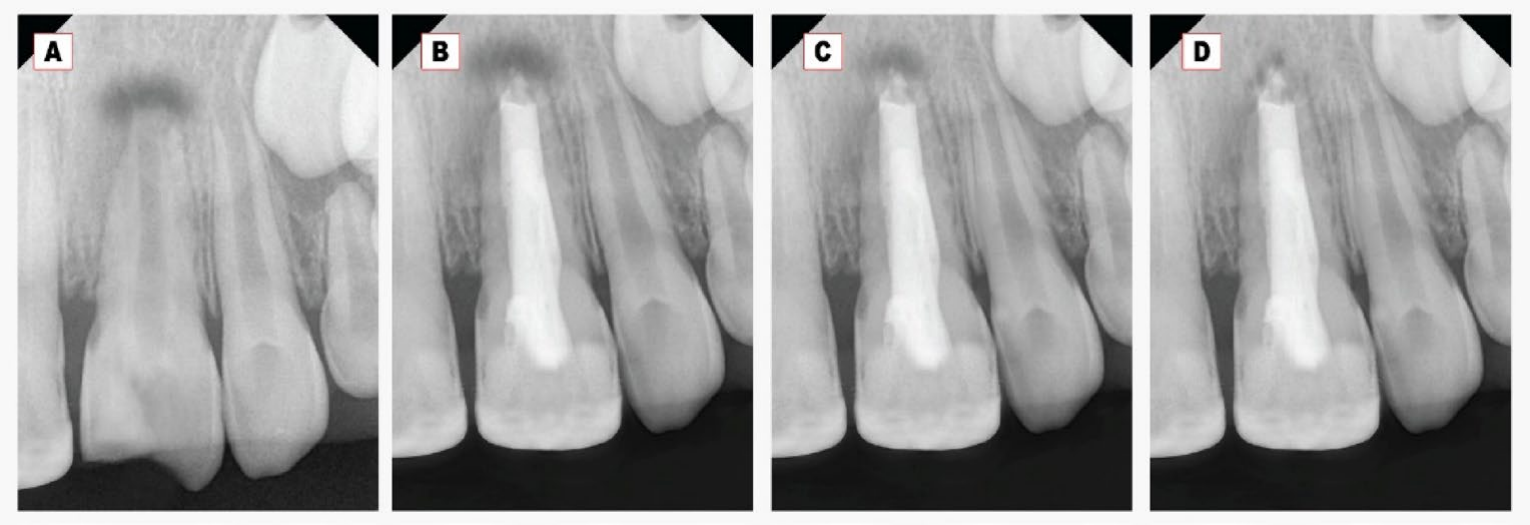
A: Preoperative intraoral peri-apical (IOPA) radiograph of upper left permanent central incisor. B: Immediate IOPA radiograph after MTA plug and gutta-percha obturation. C-D; Post-IOPA radiographs at 6 and 12 months.
